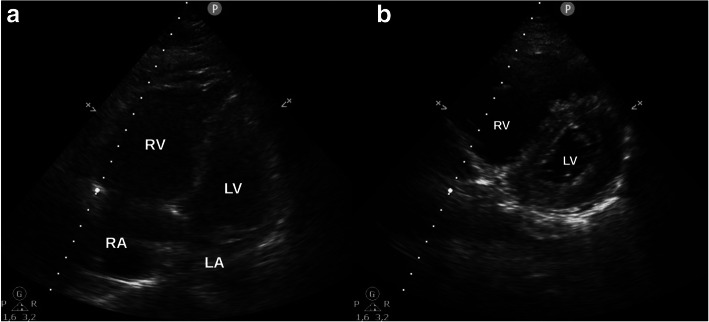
a Apical four-chamber view showed severe RV dilatation and b parasternal short axis view revealed flattened interventricular septum (LA = left atrium, RA = right atrium, LV = left ventricle, RV = right ventricle)
